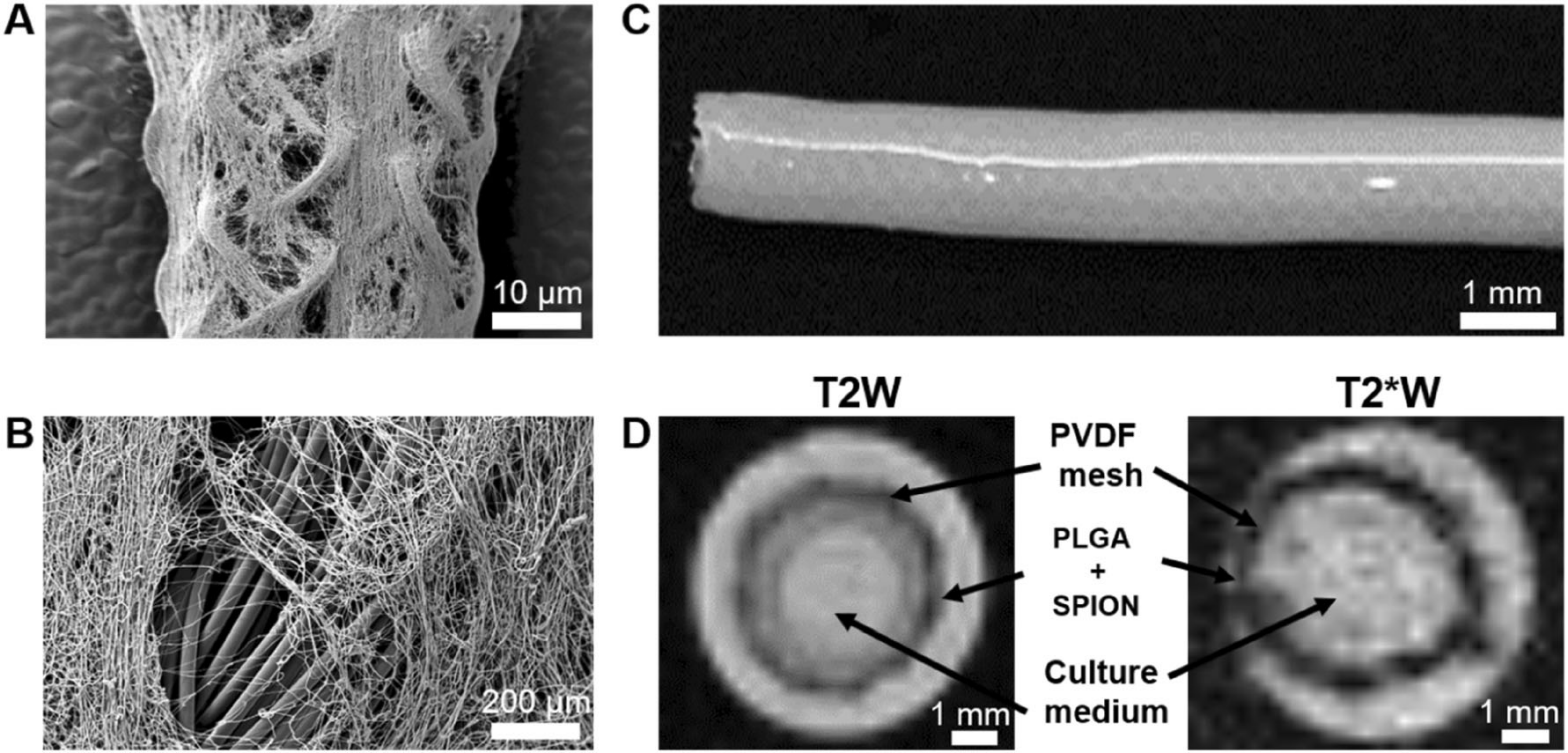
Electrospun PLGA coated TEVG. A, B SEM images of the PLGA and SPION coating on the textile mesh. C TEVG after molding. D MR images showing the lumen area and the coating upon the textile mesh
